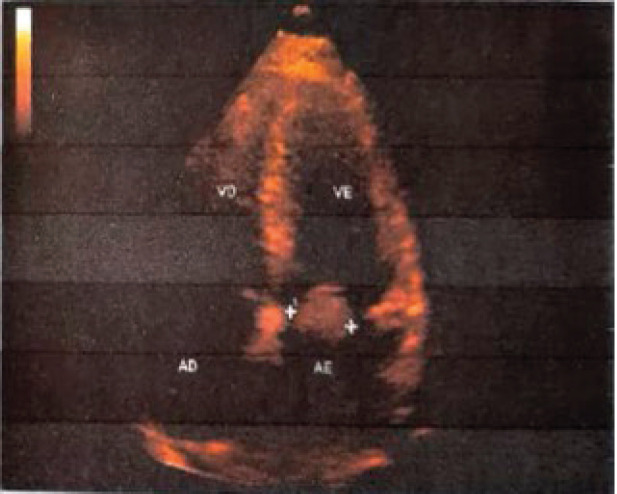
Echocardiogram with transthoracic Doppler showing a mass in the region. Source: Image provided by the patient.
